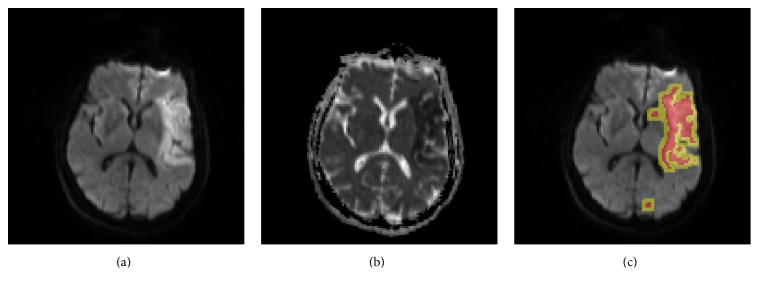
From (a) to (c), an axial slice of DWI and ADC and the corresponding ROIs for detecting infarction overlaid in color on the DWI, where regions in red are initial ROIs modified from [22] while those in yellow are from morphological dilation.
